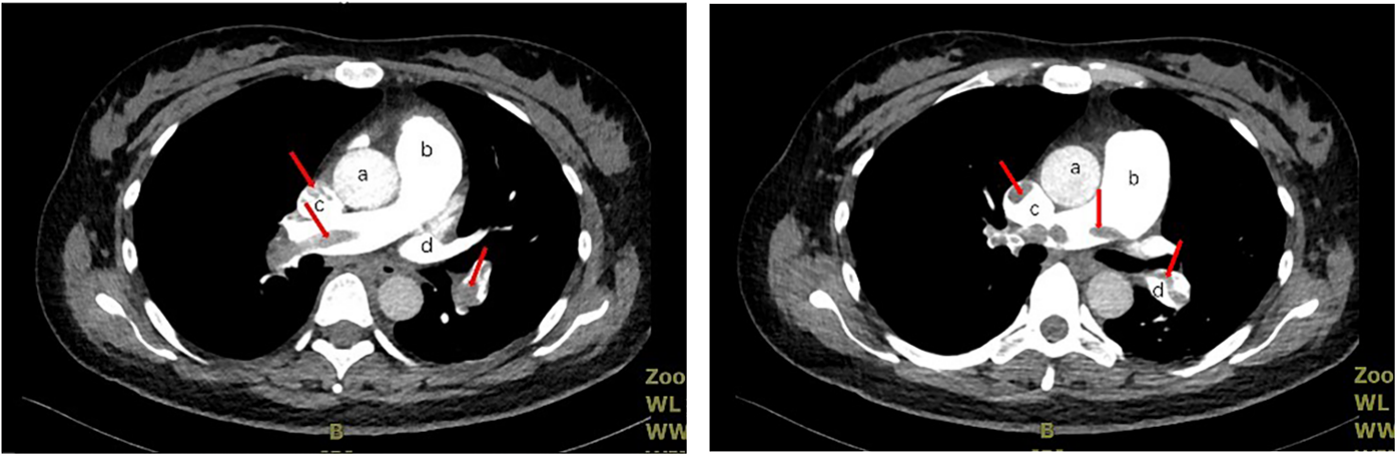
Pulmonary artery CTA image, a, aorta; b, pulmonary artery trunk; c, right pulmonary artery; d, left pulmonary artery (the red arrow indicates multiple embolisms in the left and right pulmonary arteries and pulmonary artery trunks).
